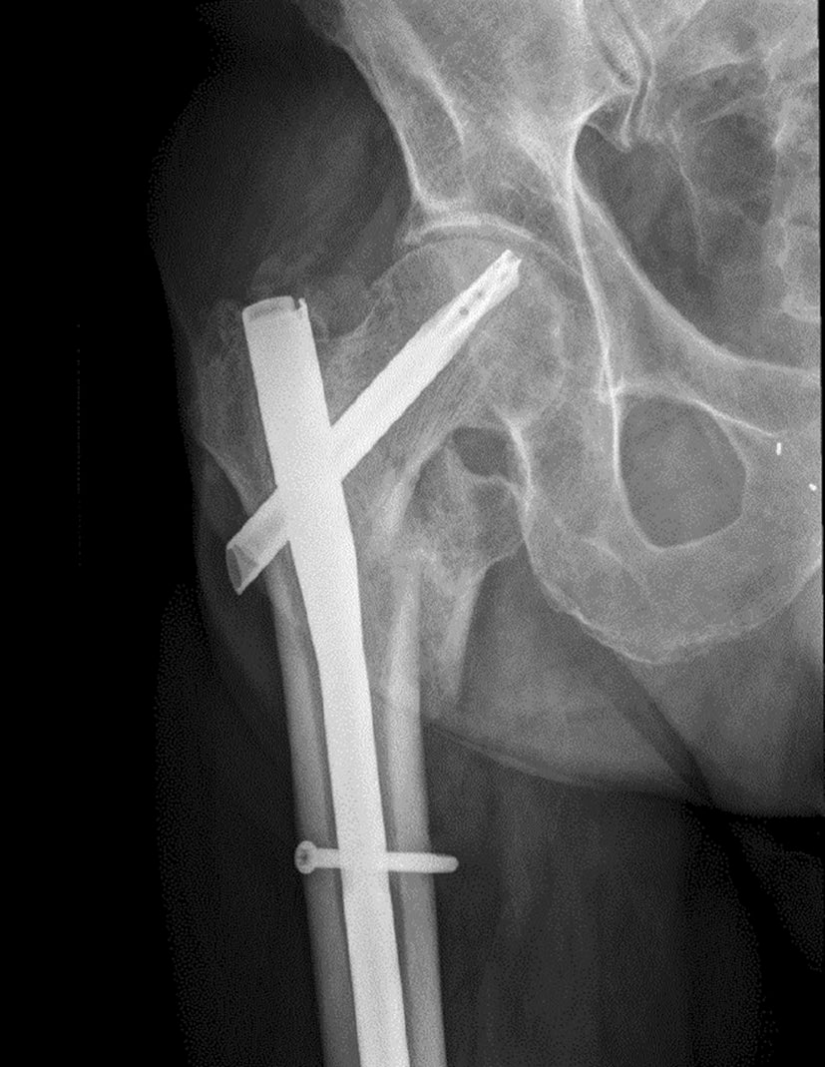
X-ray of a patient with an imminent “Cut-through"-situation
